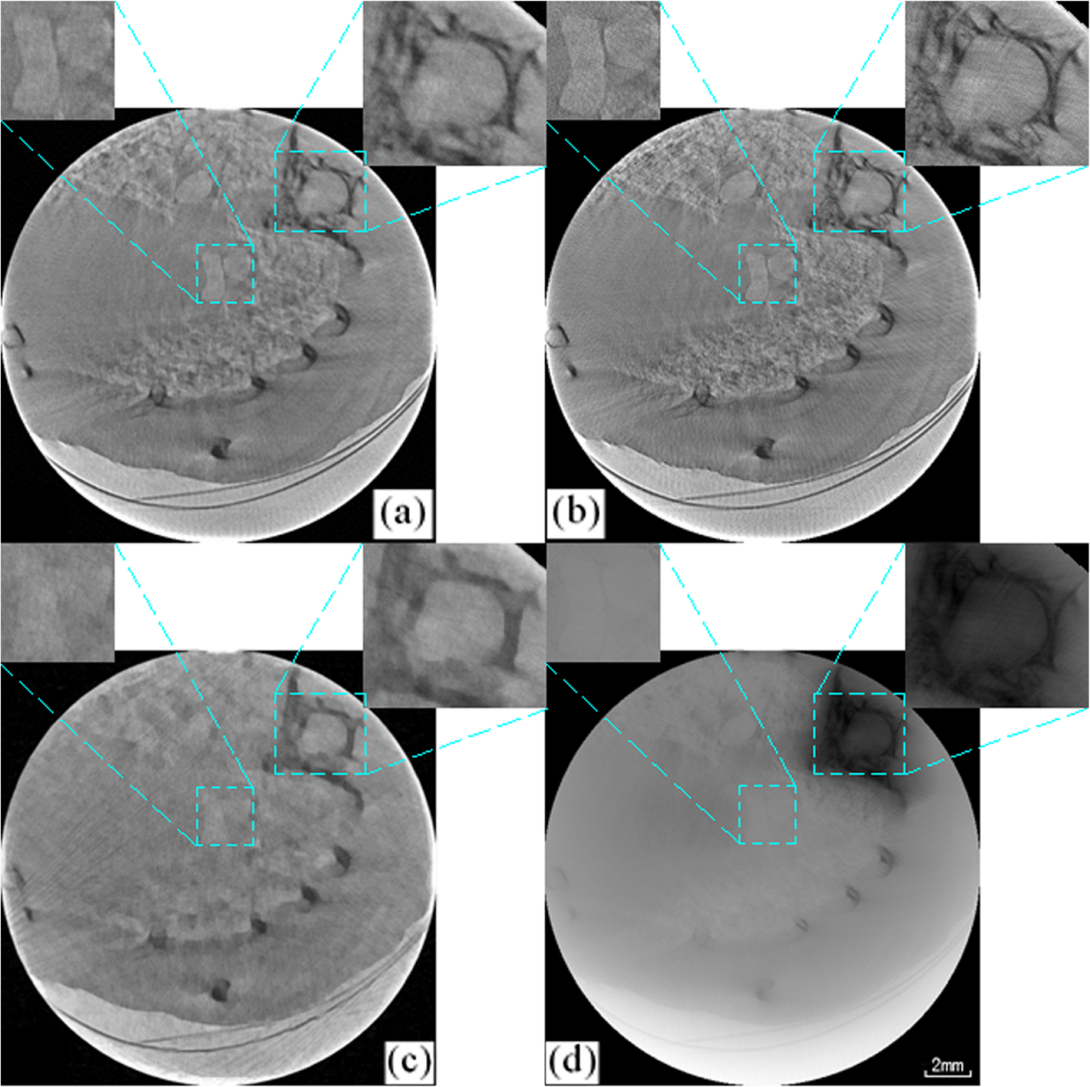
Alignment results for two images at different angles, 0° and 1. The CT reconstructed image of the 70th slice using (a) the CS-based method from 60 projection images, (b) the CS-based method from 180 projection images, (c) the CS-based method from 30 projection images, and (d) the FBP method from 180 projection images.
